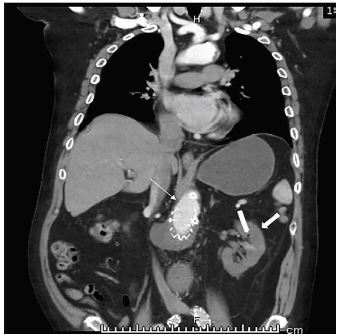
Computed tomography with contrast in coronal view demonstrating thrombosed endoluminal aortic stent (thin arrow) and infarcted left kidney (thick arrow) with intraparenchymal air (tab).
